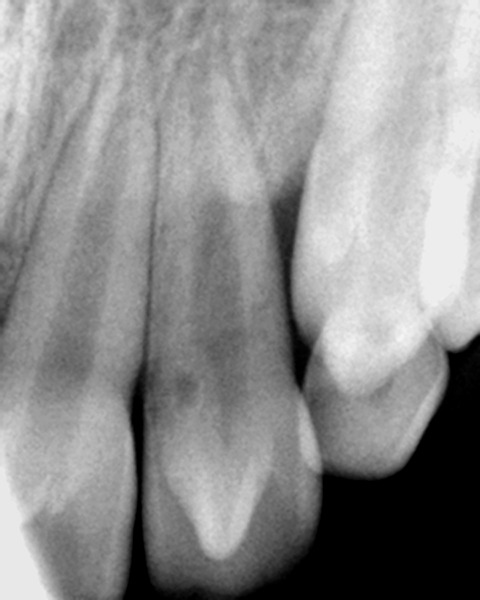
Periapical radiograph showing dens evaginatus on 22
